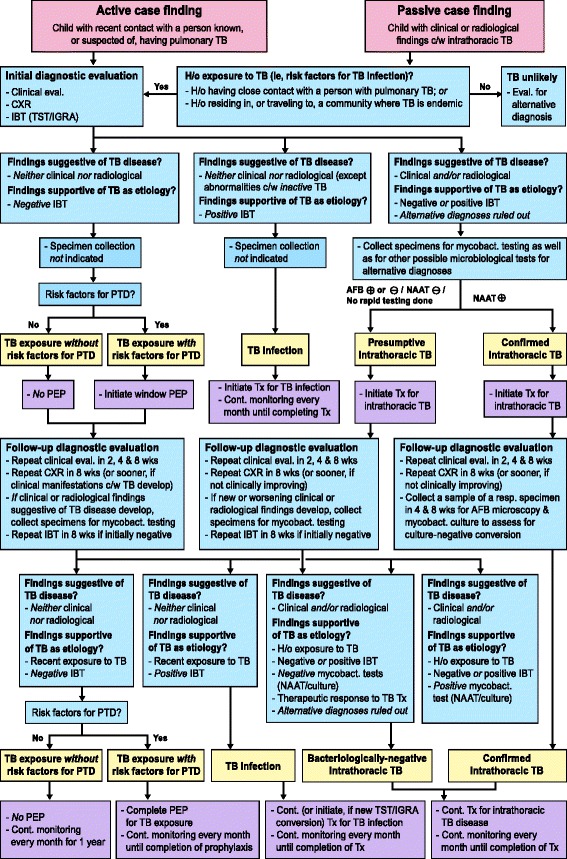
Proposed diagnostic and management algorithm for a child with recent exposure to, or with clinical or radiological findings compatible with TB. AFB: acid-fast bacilli testing; Cont.: continue; c/w: compatible with; CXR: chest radiography; eval.: evaluation H/o: history of; IBT: immune-based test IGRA: interferon-gamma release assay; mycobact.: mycobacterial; NAAT: nucleic acid amplification test; PEP: post-exposure prophylaxis; PTD: progression to TB disease; TB: tuberculosis; TST: tuberculin skin test; Tx: treatment; wks: weeks
